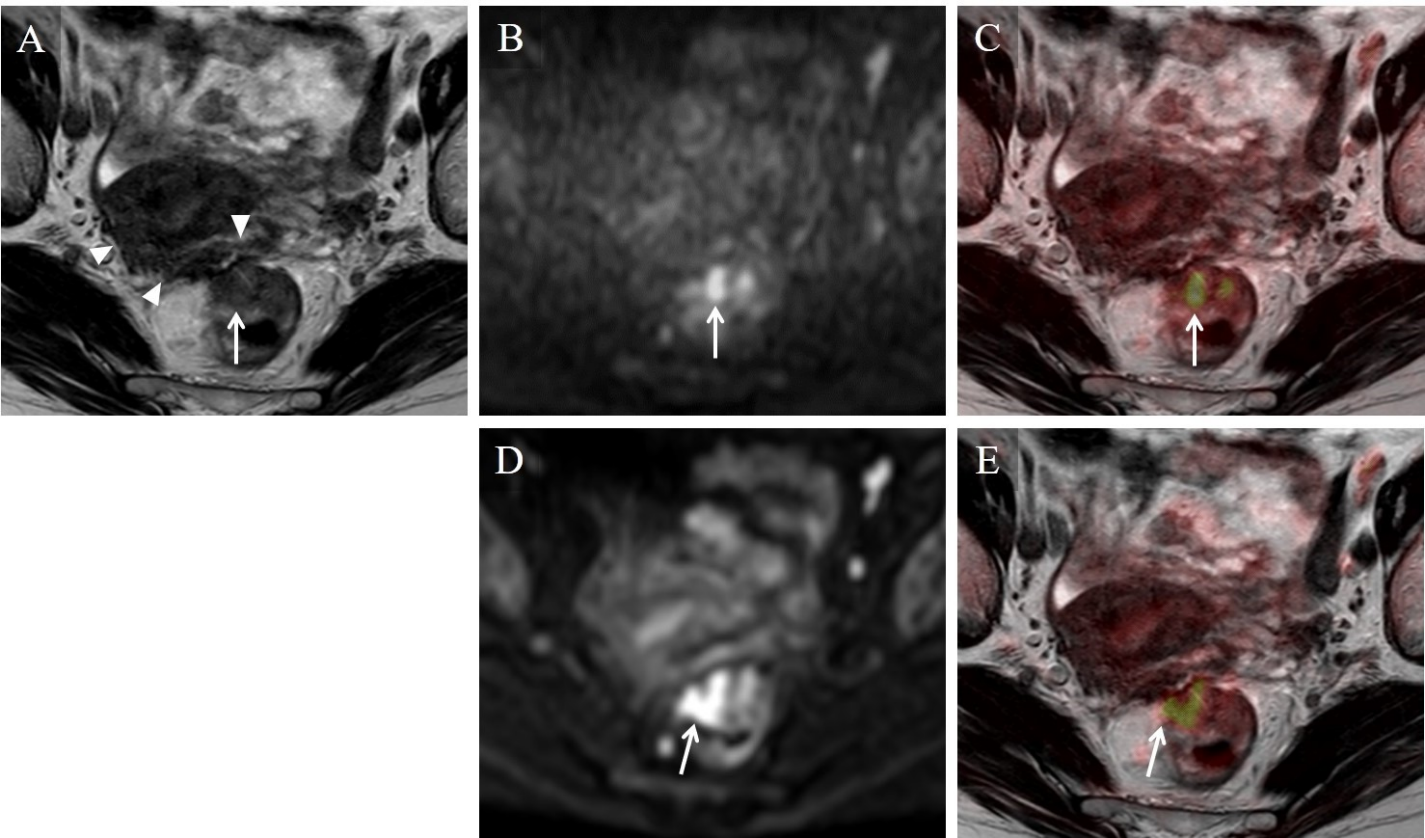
Stage T2 rectal carcinoma in a 75-year-old woman. On the T2WI image (A), a low intensity area extended from the mesorectal fascia into the surface of the uterus (arrow-head). Five readers misdiagnosed the stage as T4a or T4b with T2WI alone. This area did not show high intensity on the DWI image, and was considered as fibrosis. A high intensity lesion was seen on TSE-DWI (B) and EPI-DWI (D) image (arrow). On the fusion image of TSE-DWI (C), 7 readers accurately diagnosed the stage as T2. On the fusion image of EPI-DWI (E), an area of diffusion restriction was shown beyond the muscularis propria (arrow) probably due to image distortion, and the stage was misdiagnosed as T3.
